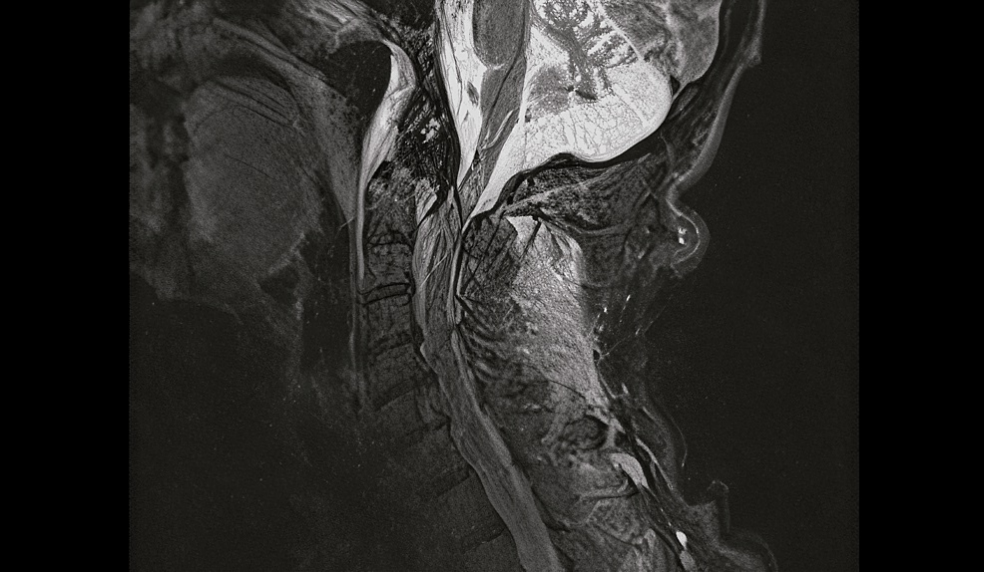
Sagittal T2-weighted magnetic resonance imaging of the cervical spine. Type II odontoid fracture with posterior dislocation causing cord compression.
